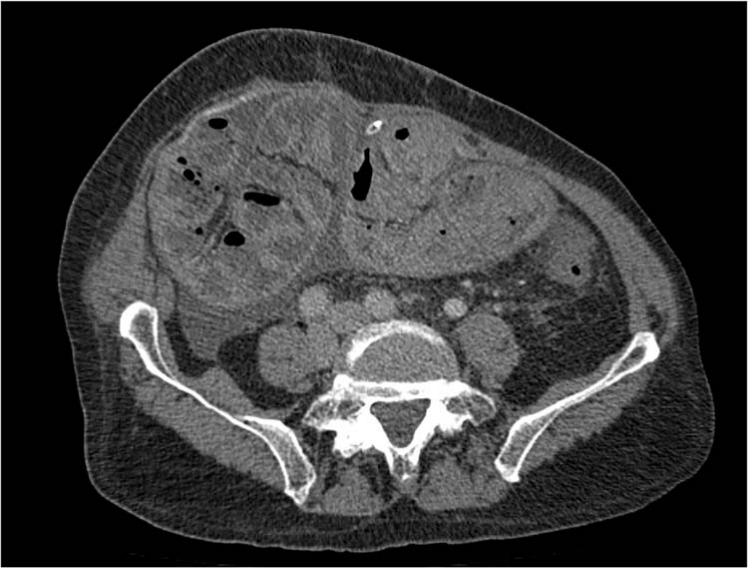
Portal phase transversal section image on CT showing loops wrapped in a membrane-like structure and thickened peritoneum.
